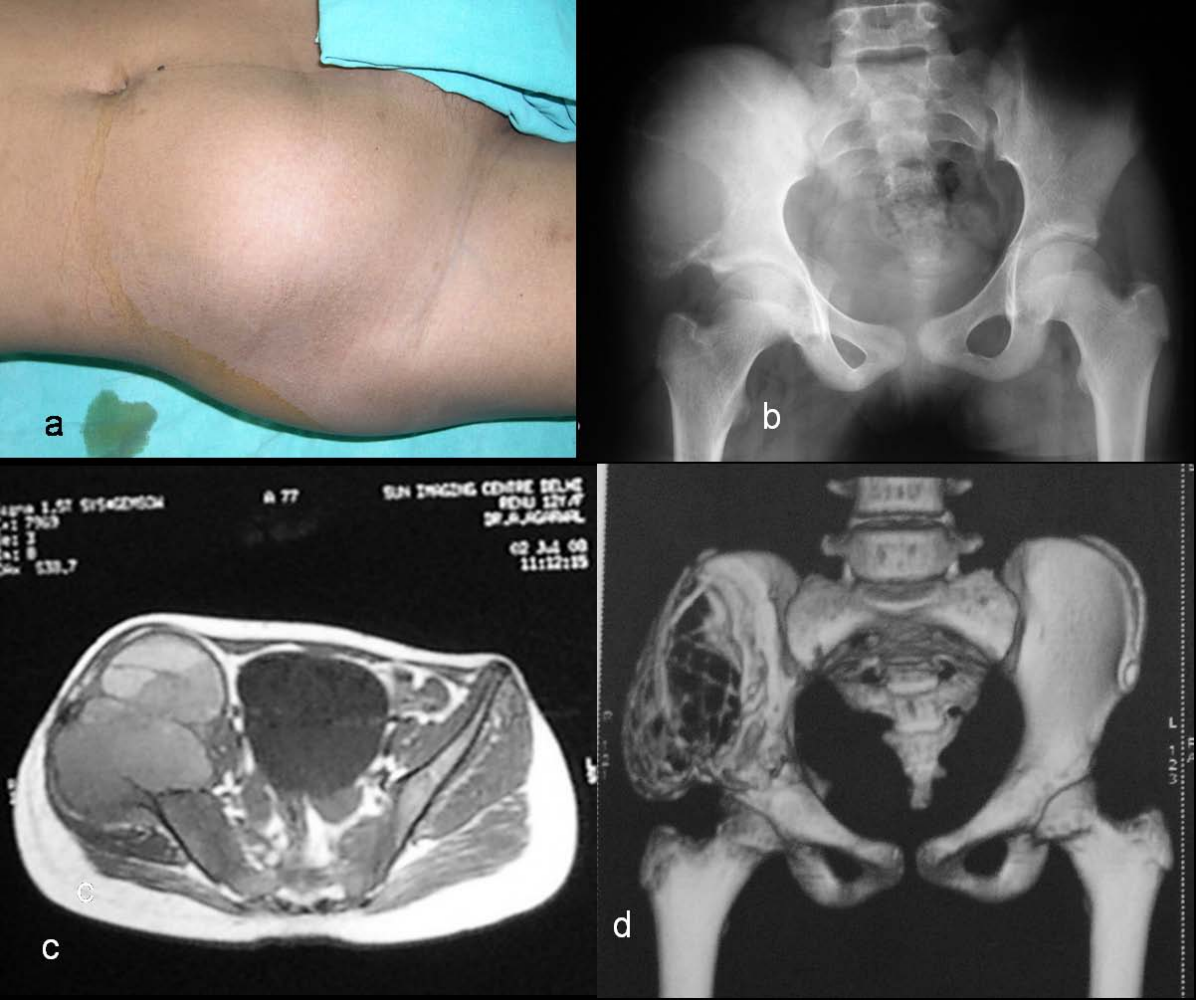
a) Pre-operative clinical photograph showing a large swelling over the right iliac region. b) Plain radiographs of right ilium showing involvement of the iliac wing. Multiple septations could be appreciated even on plain radiographs. c) MRI scan of right iliac region showing multiple fluid levels. d) 3D-CT reconstruction of the lesion showing a huge honeycomb appearance of lesion occupying almost whole of the right iliac wing with extension to superior acetabulum.
